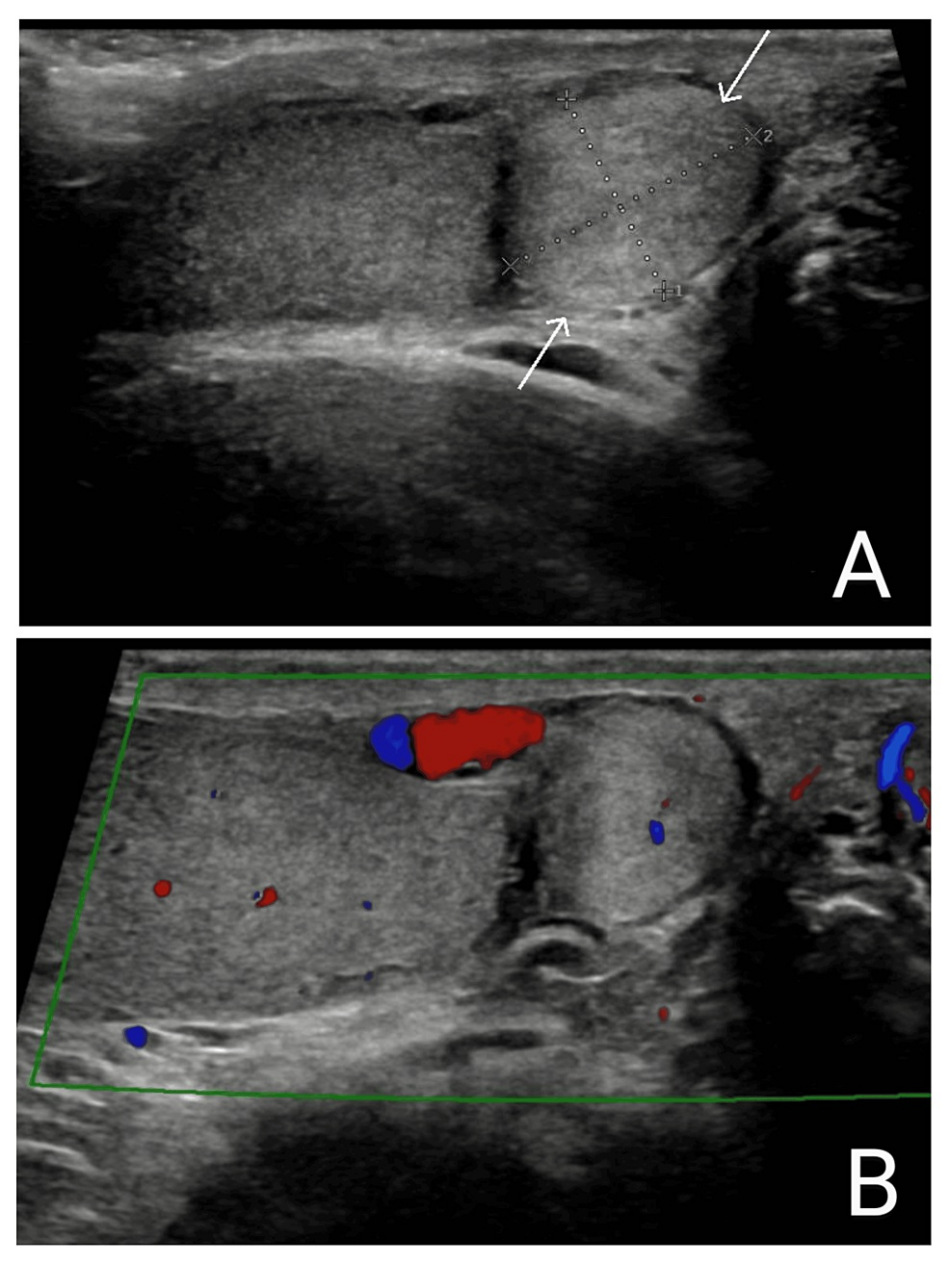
Epididymal adenomatoid tumour. Longitudinal grayscale (A) and color flow Doppler (B) ultrasounds of the scrotum reveals a well-defined solid mass (within arrows), measuring 12 x 10 mm in size and arising from the right epididymal tail without any disruption of the architecture of the testical parenchyma. The lesion showed minimal internal vascularity and slightly increased echogenicity compared to the testicular parenchyma.
